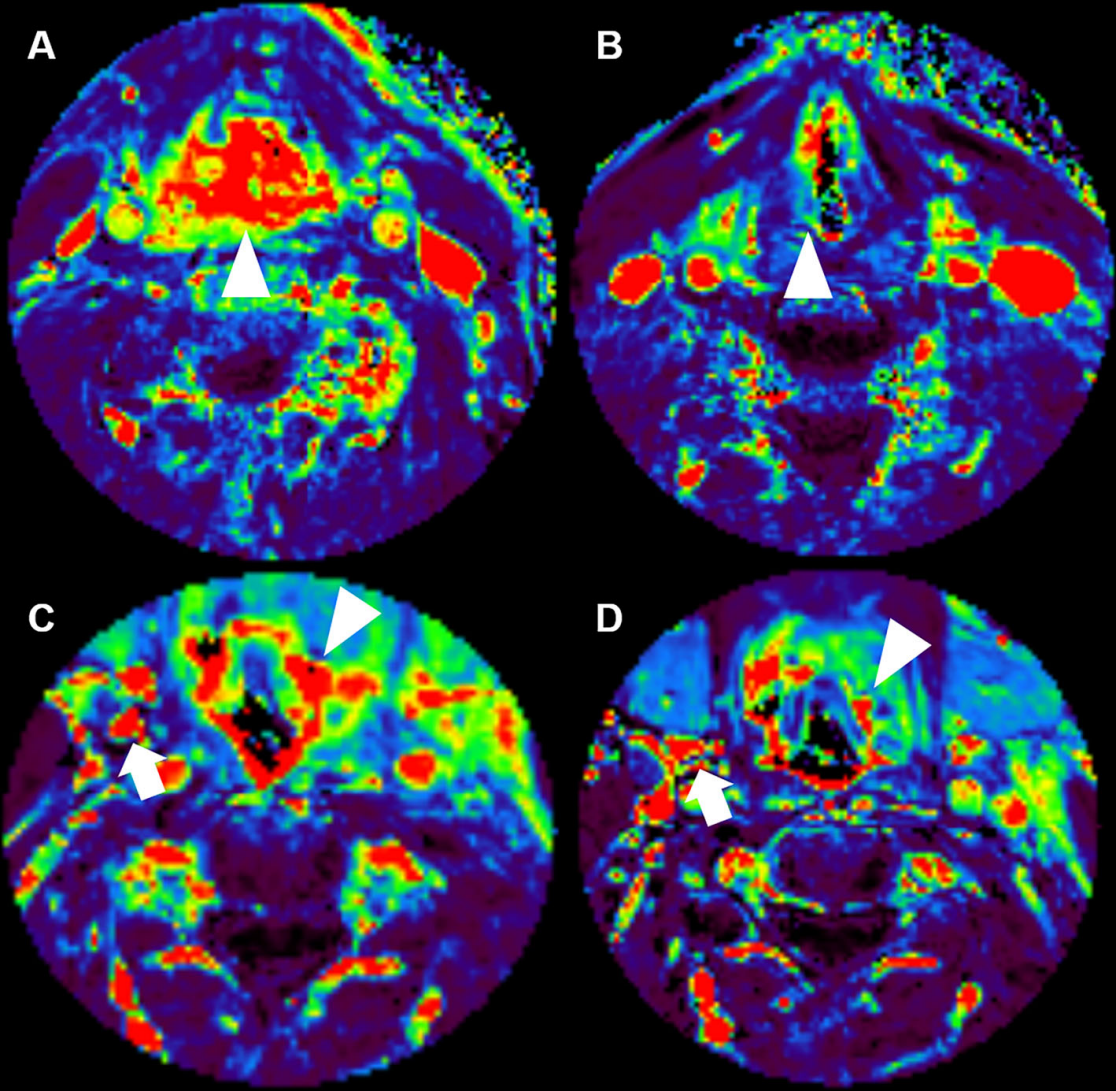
Corresponding positive enhancement integral maps of DCE MRI for the same patients as shown in , pathologic complete response (upper row) and residual tumor (lower row) at baseline (A, C) and at follow-up (B, D). The area of the primary tumor is marked with an arrowhead, lymph node metastasis is marked with an arrow.
